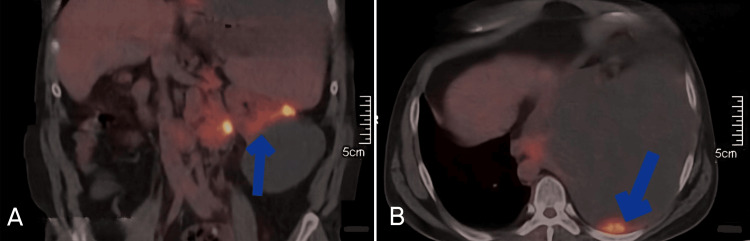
Whole-body PET-CT scan showing: (A) Metabolically active left renal mass (blue arrow), (B) Metabolically active soft tissue mass in the left pleura (blue arrow). PET-CT: Positron emission tomography-computed tomography.
